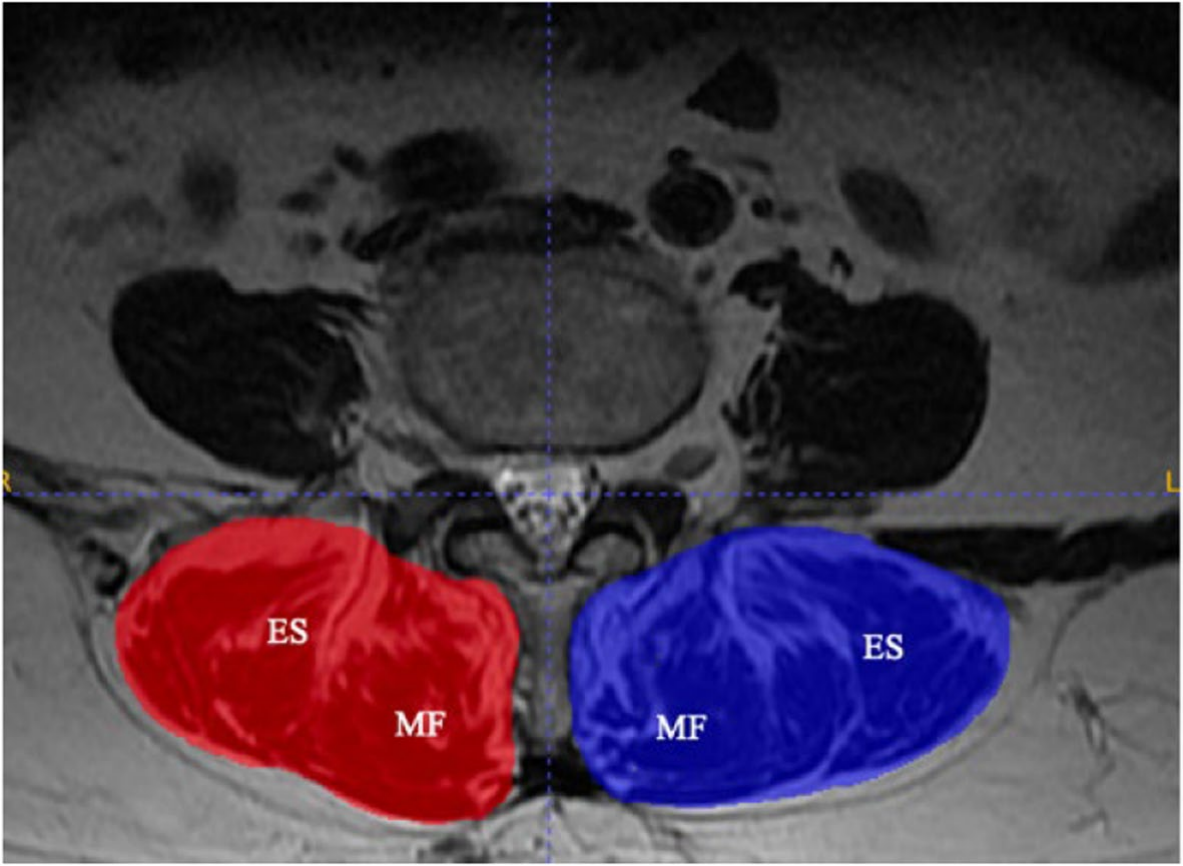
Bilateral manual segmentation of psoas and paraspinal muscles (erector spinae (ES) and multifidus (MF)) on axial T2-weighted MRI
